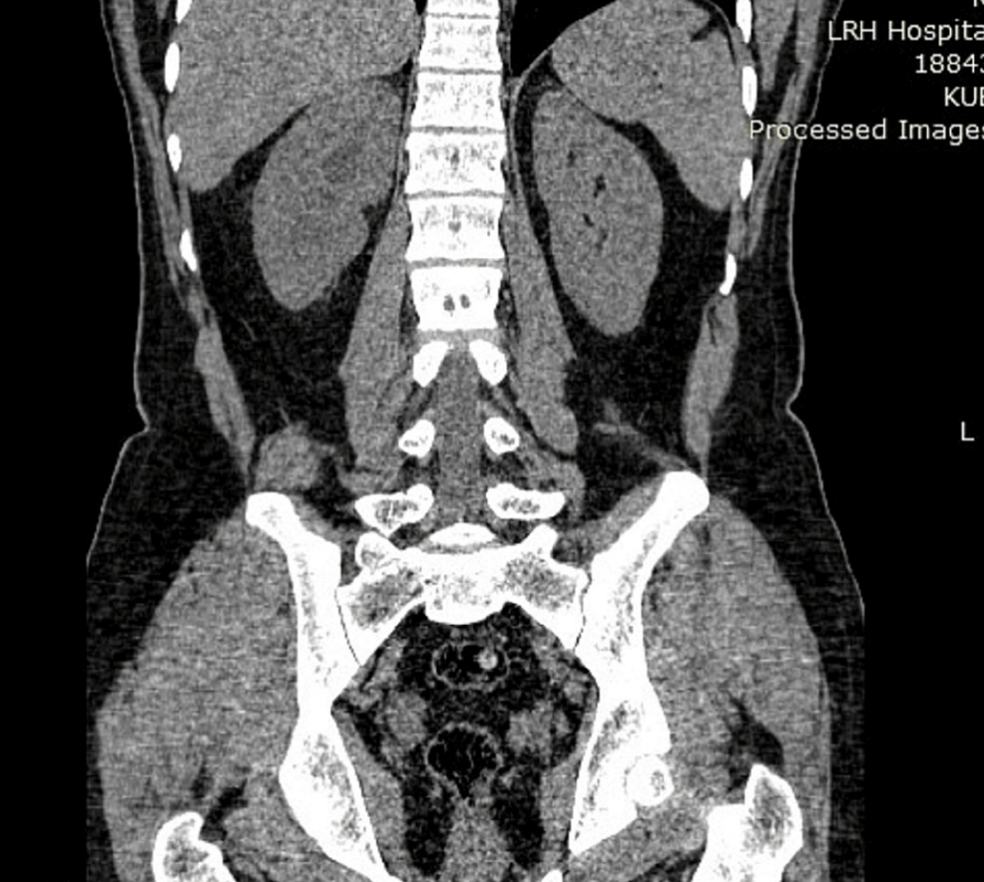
Postoperative CT KUB image (stone clearance achieved using Pneumatic Swiss LithoClast). CT KUB = computed tomography of the kidneys, ureters, and bladder
